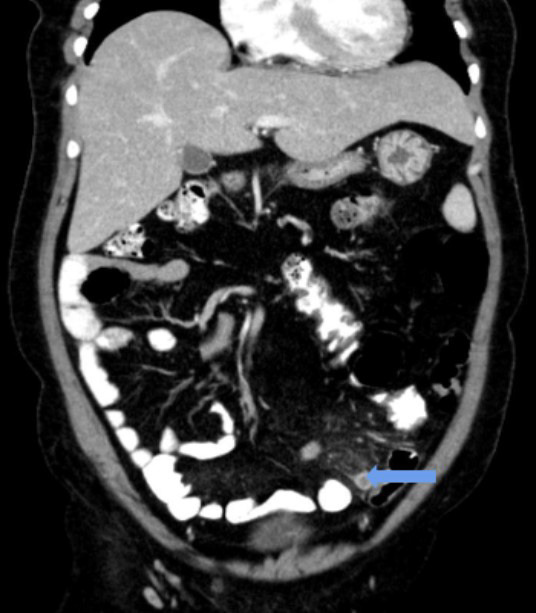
Coronal image of contrast-enhanced CT scan of the abdomen shows left-sided appendicitis (block arrow) with peri-appendiceal fat stranding.
